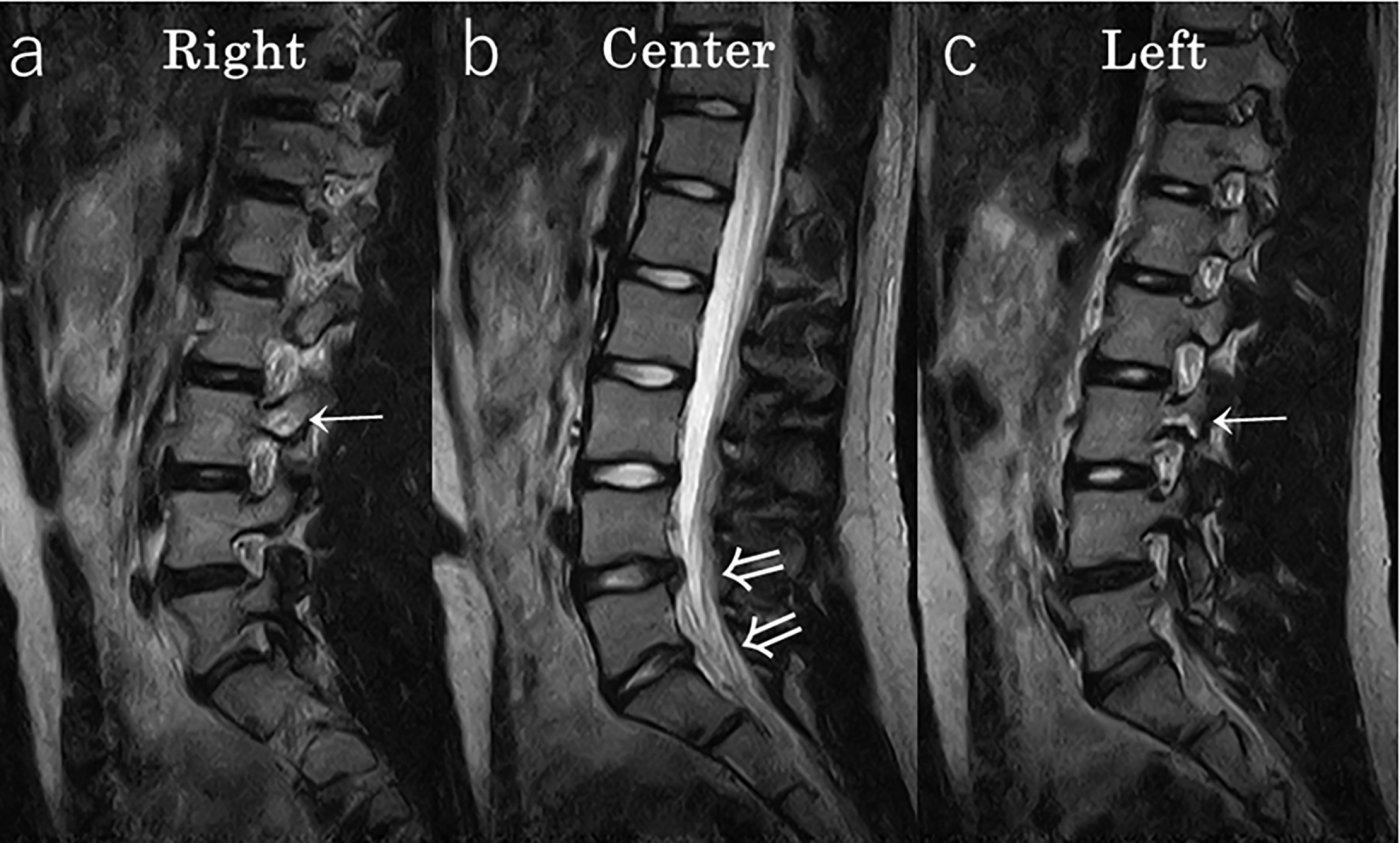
Representative case #1 MRI of a 14 year-old girl in 2016. Lumbar spondylolysis was noted bilaterally at the L3 level (a,c: →), disc protrusion was noted at the L4/5 level, and disc degeneration was found at the L4/5 and L5/S1 levels (b: ⇒).
